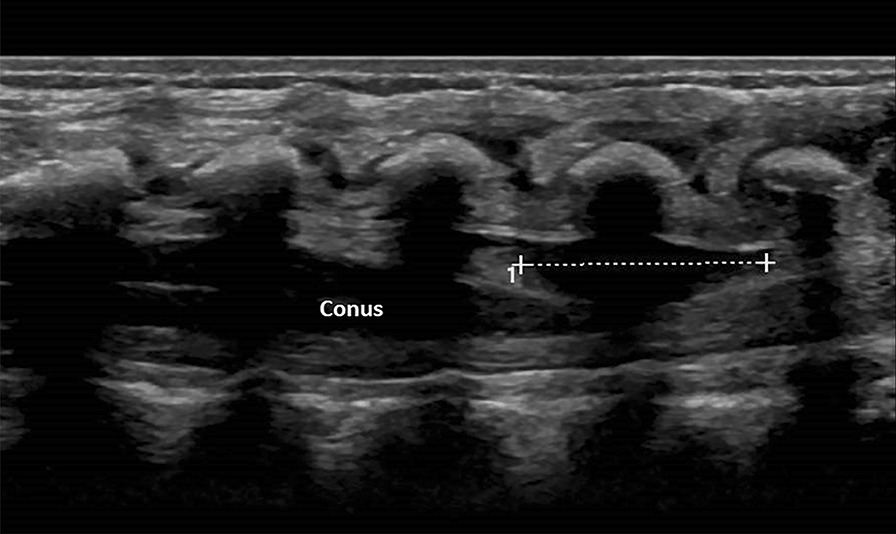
Sagittal ultrasound of lumbar spine at 1 day of age. The conus ends at L2. Note the oblong anechoic cyst at the conofilar junction dorsal to the conus medullaris
